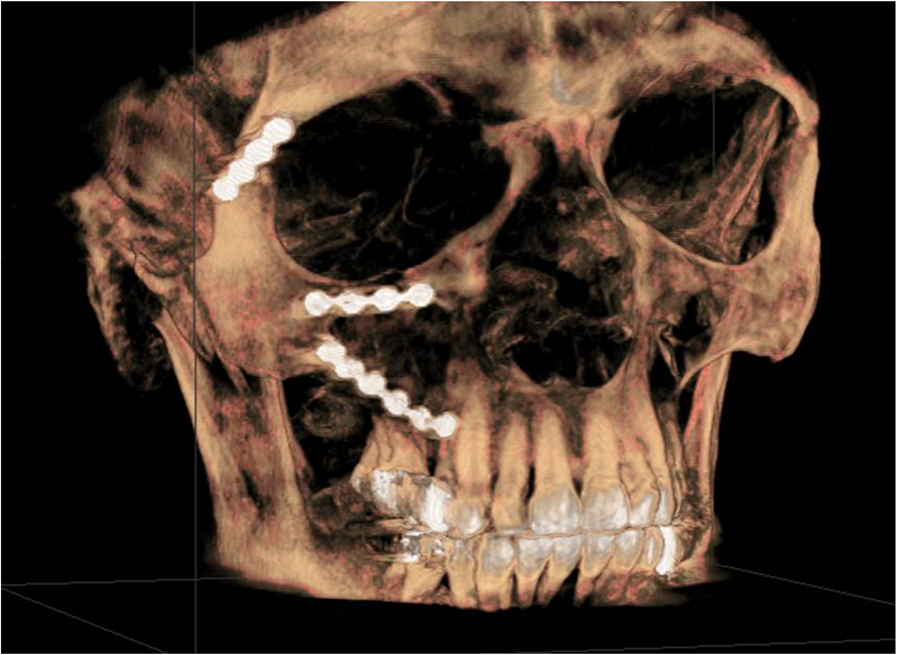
Three-dimensional reconstruction of postoperative cone beam computed tomography after osteosynthesis of a right-side zygomatical fracture, along the frontozygomatic suture, infraorbital margin and zygomatico maxillary buttress.
